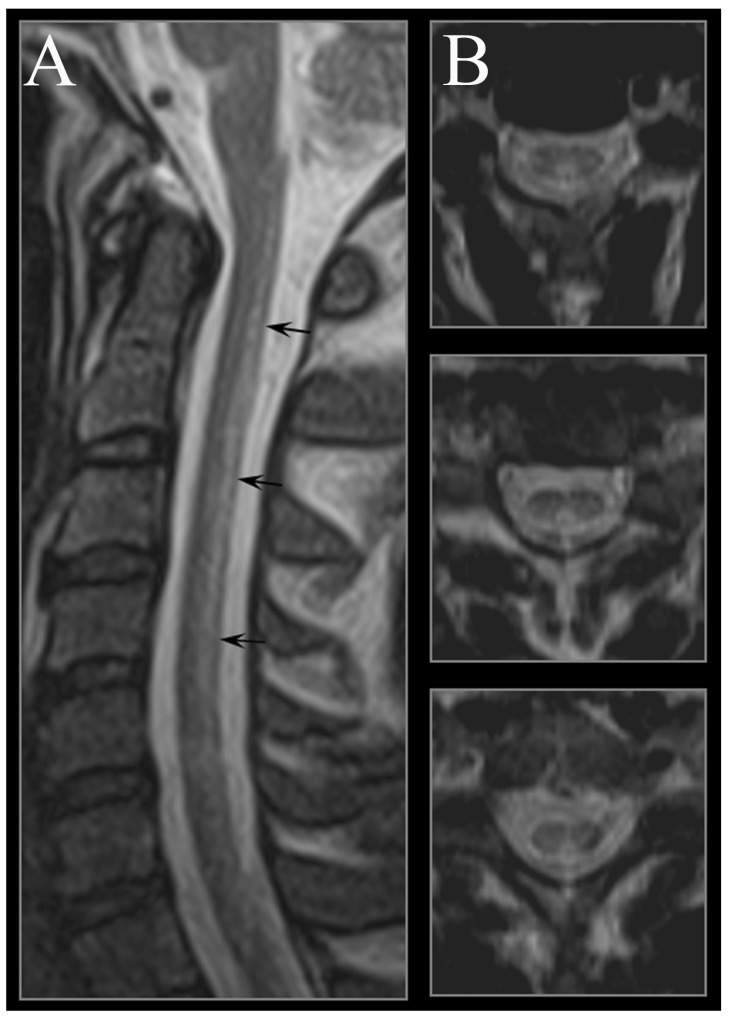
Cervical spinal cord MRI in a 49-year-old male presenting with subacute combined degeneration due to a deficit of B12. (A) The midsagittal T2 weighted image shows linear hyperintensity in the posterior portion of the cervical tract of the spinal cord (black arrows). (B) Axial T2 weighted images reveal the selective involvement of the posterior columns.
